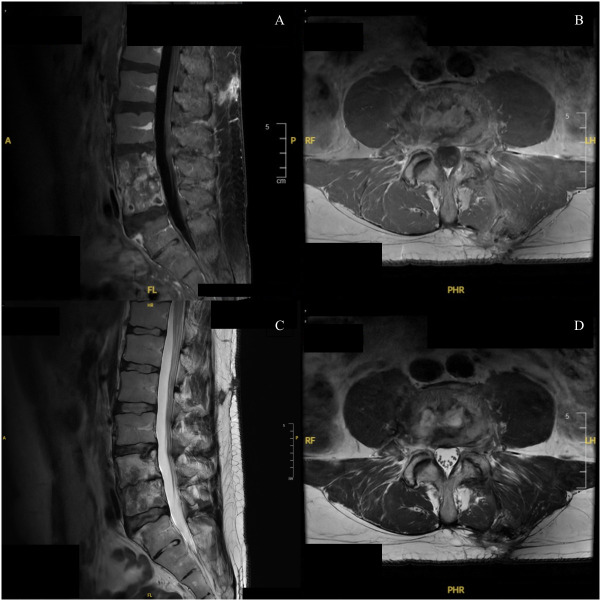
Latest follow-up MRI showing considerable improvement. Repeat MRI at the latest follow-up (Day 235) demonstrated marked improvement in the previously identified paraspinal and bilateral psoas abscesses, with decreased edema and enhancement involving the L4–L5 vertebral bodies, L4–L5 disc, and anterior S1 vertebral body. Epidural enhancement posterior to L4 and L5 also improved significantly, although residual enhancement persisted in the left greater than right L4–L5 neural foramina. (A) Sagittal T1 post-contrast MRI, (B) axial T1 post-contrast MRI, (C) sagittal T2 image, and (D) axial T2 image. Alt text: Follow-up lumbar spine MRI (Day 235) demonstrating marked improvement of prior L4–L5 spondylodiscitis, with resolution of paraspinal and psoas abscesses, decreased vertebral and disc edema, and reduced epidural enhancement, with minimal residual enhancement in the L4–L5 neural foramina.
